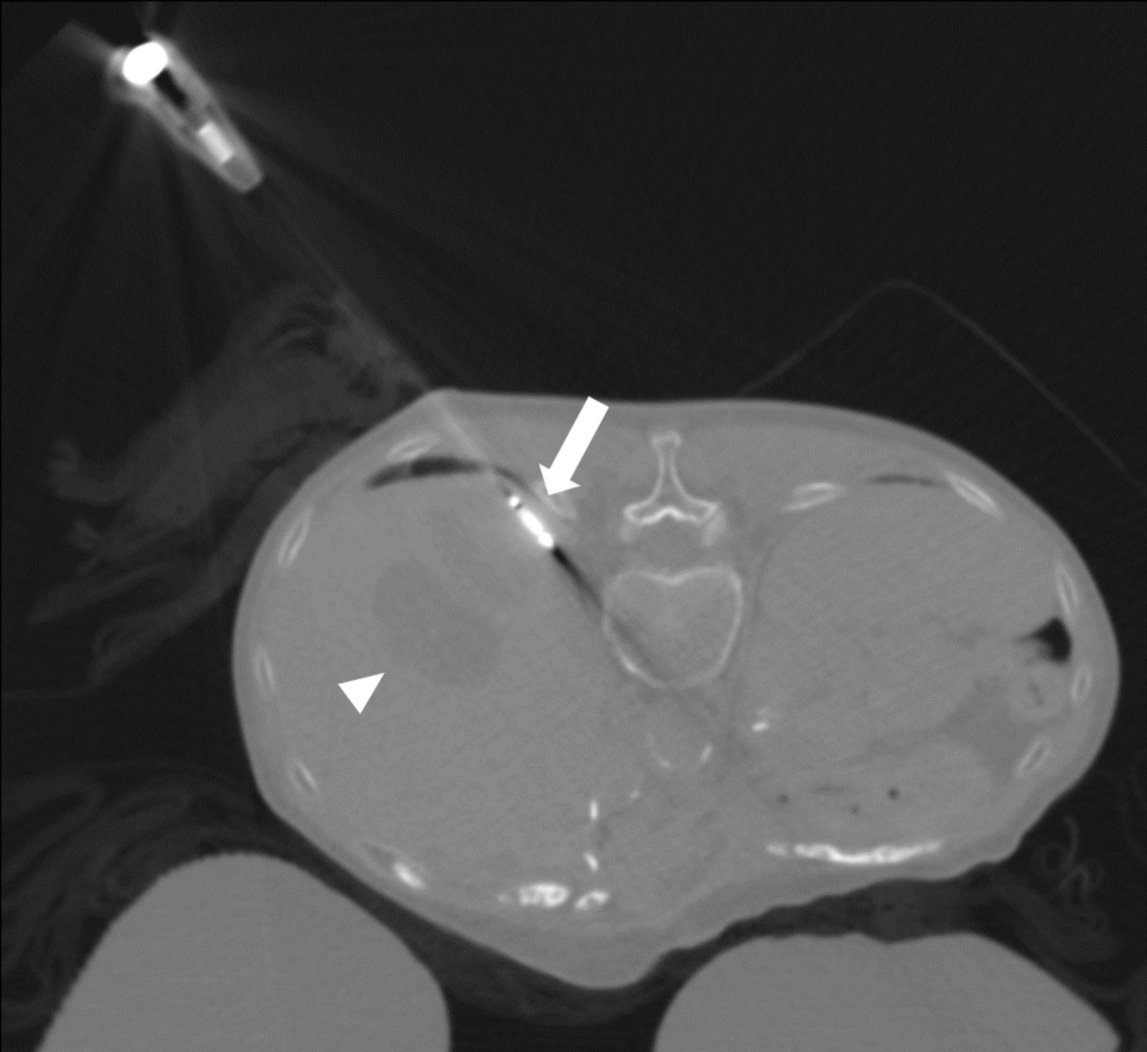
Intraprocedural ablation axial CT image Microwave ablation antenna positioned in the subdiaphragmatic nodular component of the embolization cavity (arrow). Anterior to this is a partially visualized simple cyst (arrowhead). CT: computed tomography
